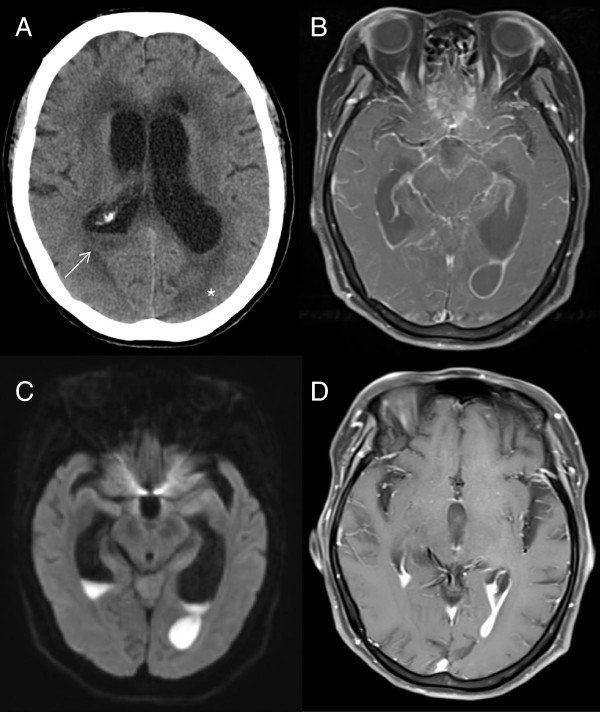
Plain computed tomography (CT) and contrast-enhanced magnetic resonance imaging (MRI) of the brain on days 6 and 30. A: Plain CT on day 6. Debris in the right lateral ventricle (white arrow) and a space-occupying lesion with surrounding edema in the left occipital lobe (asterisk) were observed. B: T1-weighted, contrast-enhanced MRI on day 6. The left occipital lesion displayed ring-like enhancement. C: Diffusion-weighted imaging on day 6. Homogenous high-intensity signaling of the lesion was observed. D: Contrast-enhanced T1-weighted imaging on day 30. The left occipital lesion dramatically decreased in size.
